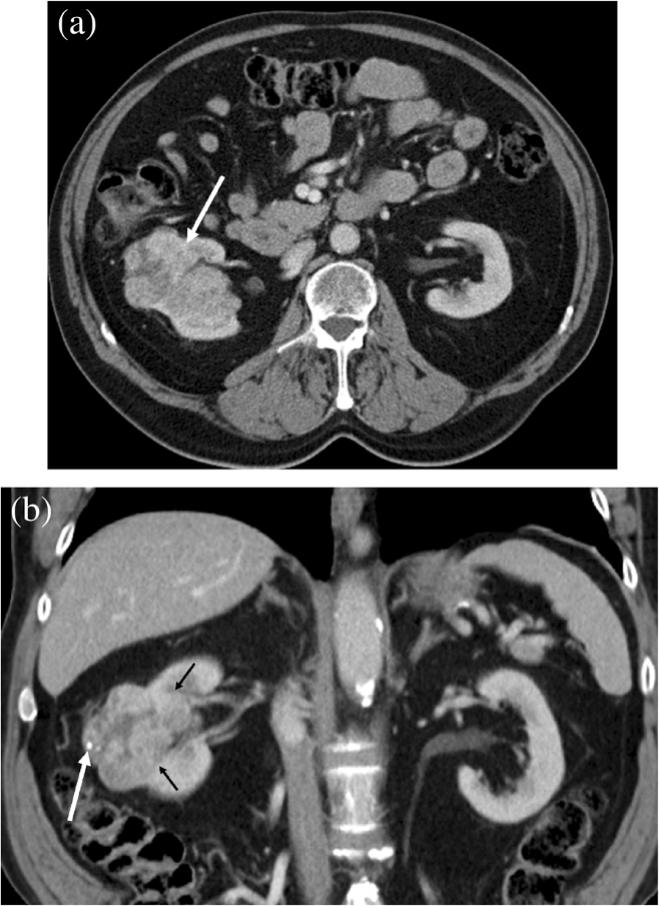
A 74-year-old male with Fuhrman grade 2 ccRCC showing lobulated tumor margin. a: Contrast-enhanced transverse CT image shows a heterogeneously enhancing lobulated mass in the right kidney (arrow). b: Coronal reformatted image shows lobulated tumor contour and well-defined tumor margin to the normal renal parenchyma (small arrows). Note calcification in the tumor (large arrow).
